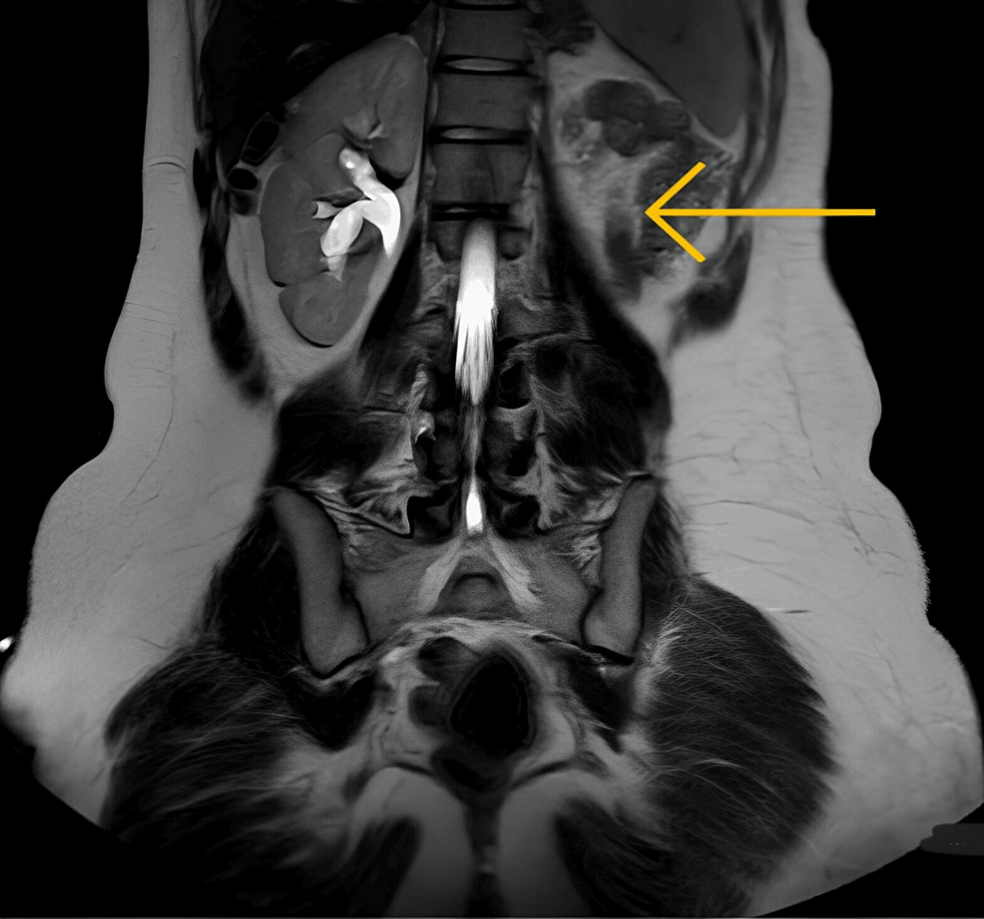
MRI showing empty left renal fossa MRI: magnetic resonance imaging
